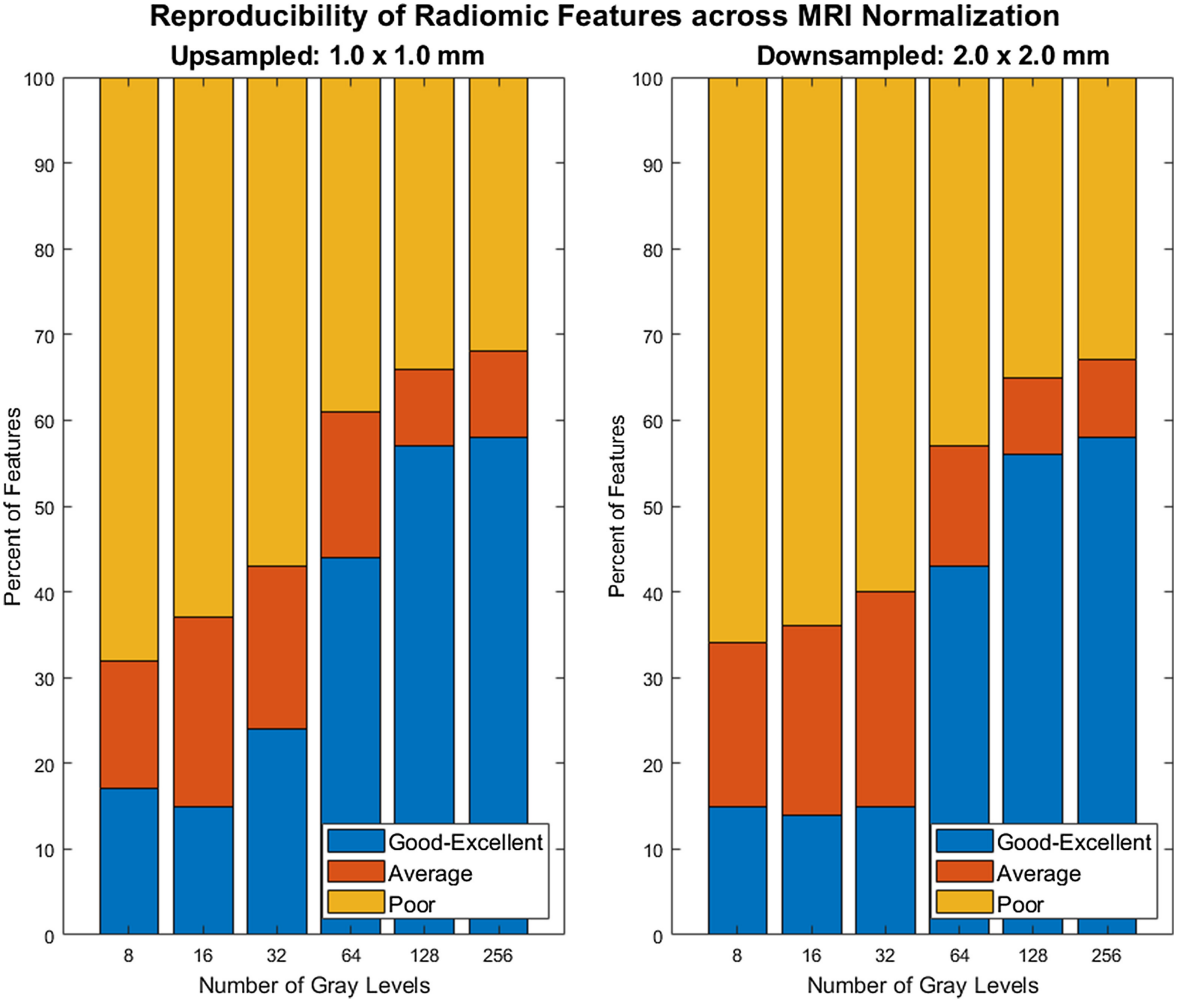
ICC scores for radiomic features (poor, moderate, and good-to-excellent) across MRI normalizations.
